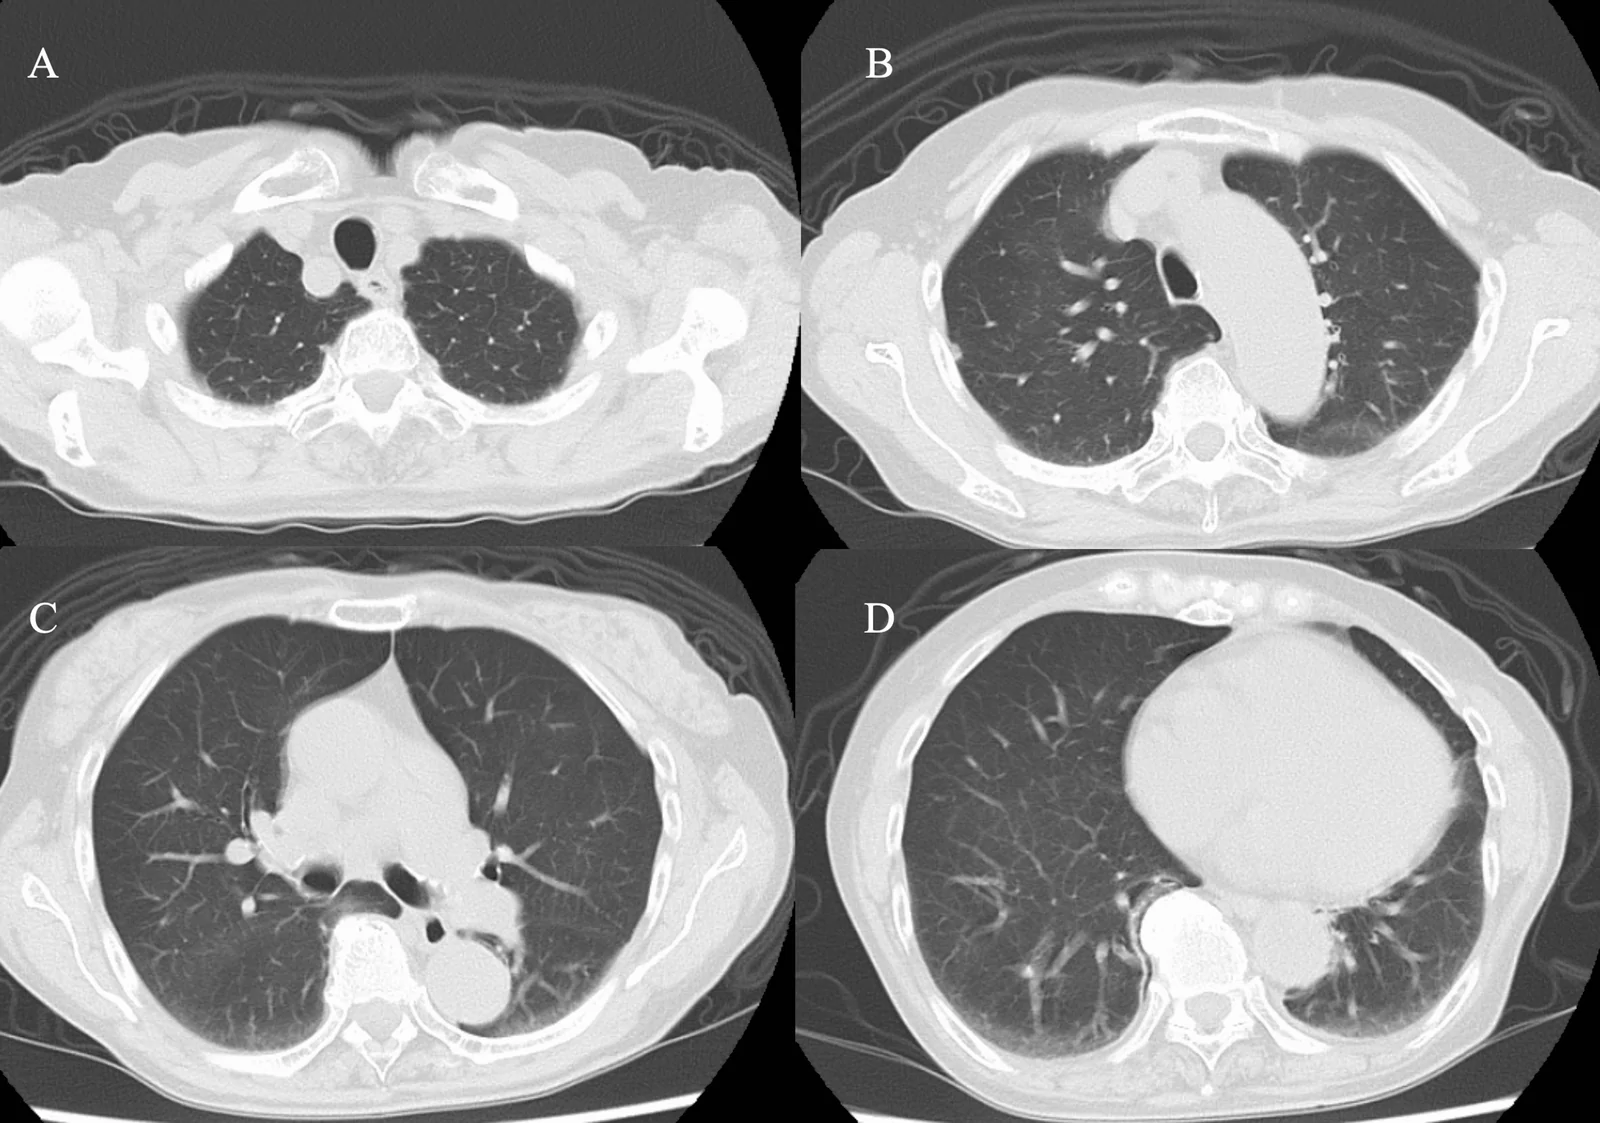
Chest CT findings CT showed no remarkable findings suggestive of pulmonary cryptococcosis in the lungs. (A) Apex. (B) Upper field. (C) Middle field. (D) Lower field CT: computed tomography
